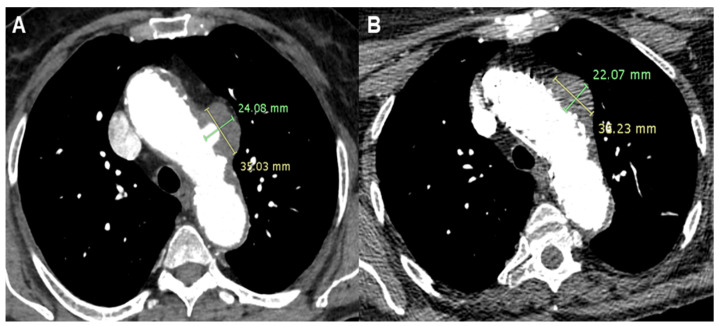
Patient 1’s Computed Tomography Angiography showing a Penetrating Aortic Ulcer (PAU) of the aortic arch (A) and the complete exclusion of the PAU after Thoracic Endovascular Aortic Repair (TEVAR) with reduction in the dimension of the pseudoaneurysm (B).
